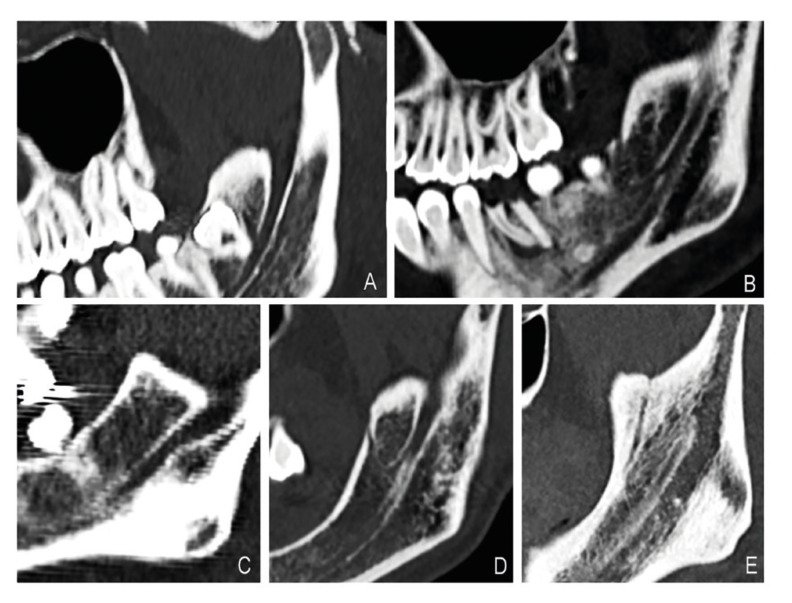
The RMC classification on the helical CT scan pictures found in this study. A: Type Ia; B: Type Ib; C: Type II; D: Type III; E: Type IV.
